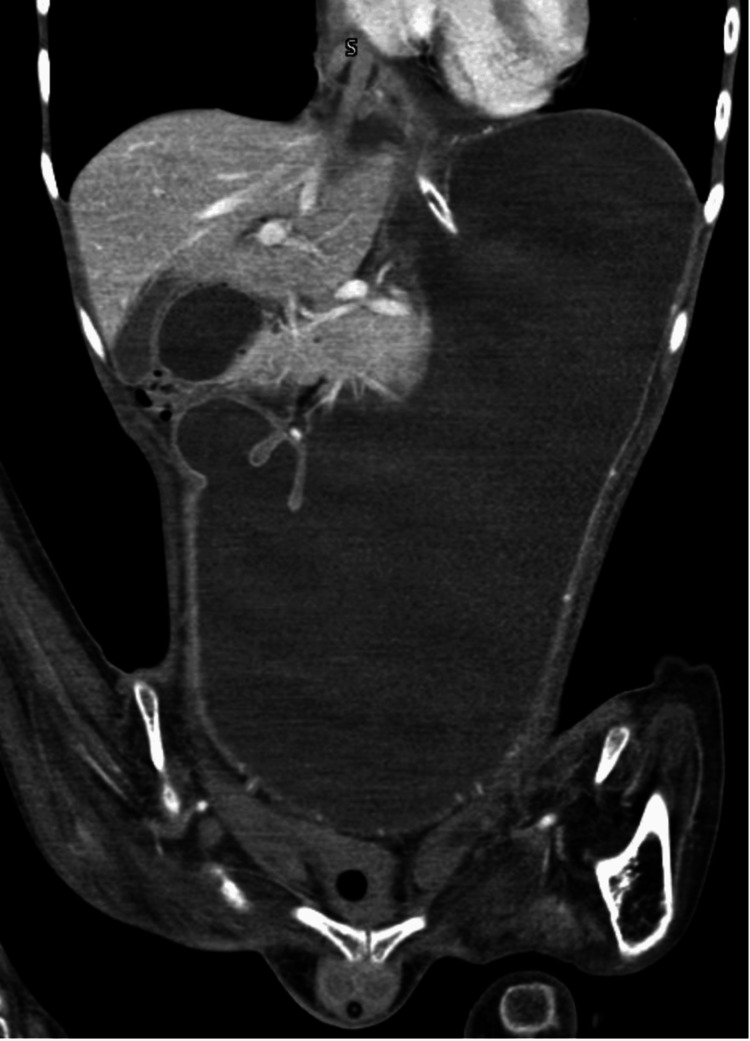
Abdominopelvic CT scan (coronal view) showing a dilated stomach and duodenum, with extension into the pelvic cavity CT: computed tomography
